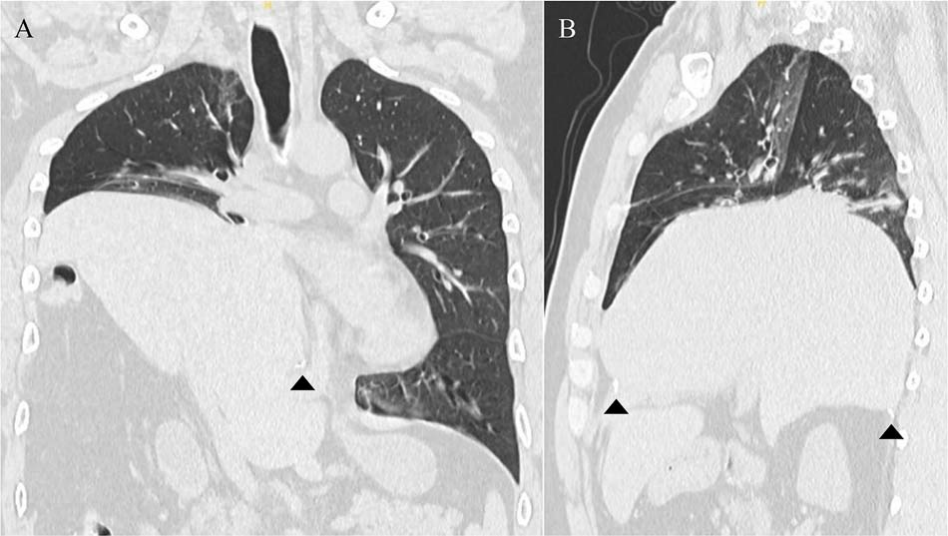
Chest computed tomography showing that the liver had prolapsed into the thoracic cavity. (A) Coronal view. (B) Sagittal view. The arrowhead indicates the edge of the torn diaphragm, including the torn staple line.
